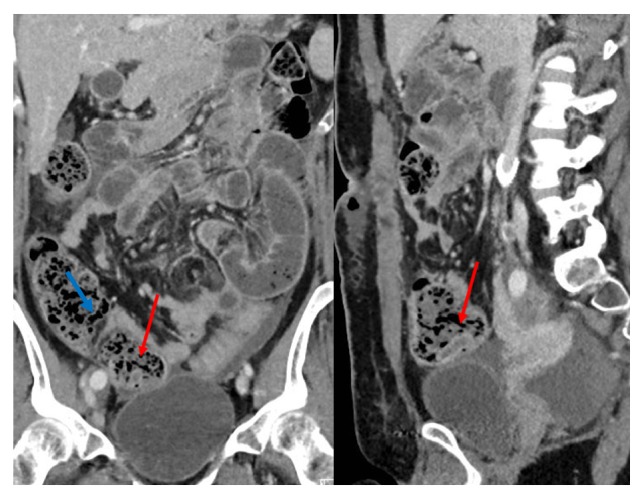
Contrast enhanced coronal and sagittal computed tomography (CT) image of the abdomen and pelvis of Patient 1 at another time reveals unchanged normal appendix (red arrow) invaginated into the cecum, now surrounded by fecal particulate matter, making detection more difficult. Note the submucosal fat from the ileocecal valve (blue arrow).
